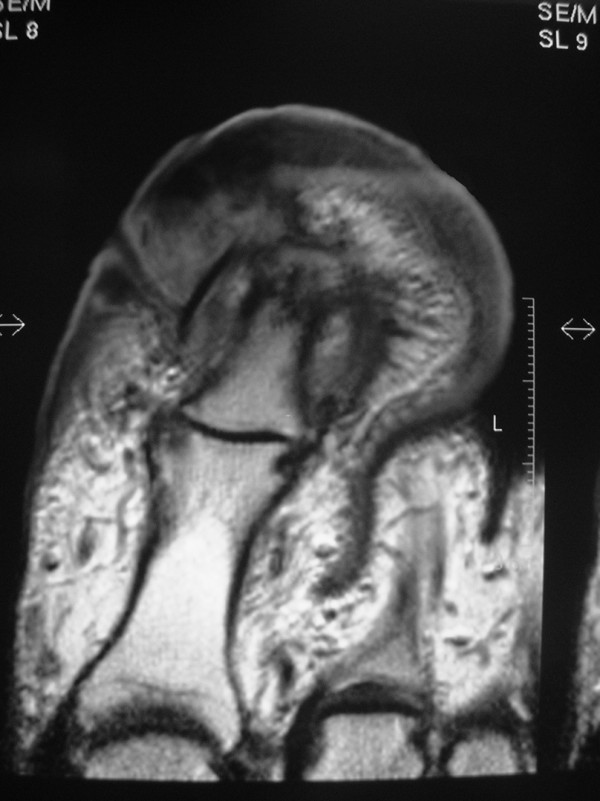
MRI scan of the great toe (case 1). As in the plain film, the end of the distal phalanx has been completely destroyed. There is signal change in the distal part of the proximal phalanx suggesting involvement of the bone more proximally.
